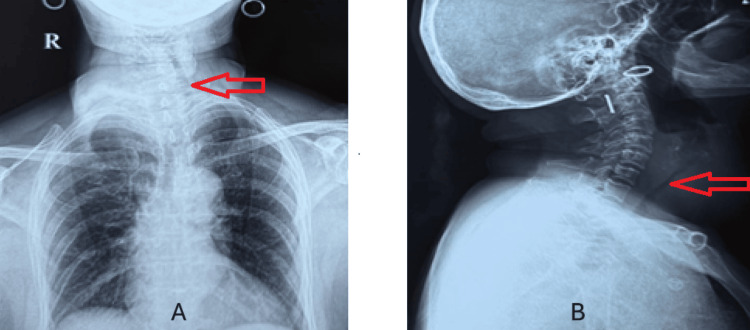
(A) Chest X-ray AP view showing tracheal deviation (red arrow). (B) X-ray neck lateral view showing tracheal compression and enlarged thyroid in the lateral view (red arrow).
